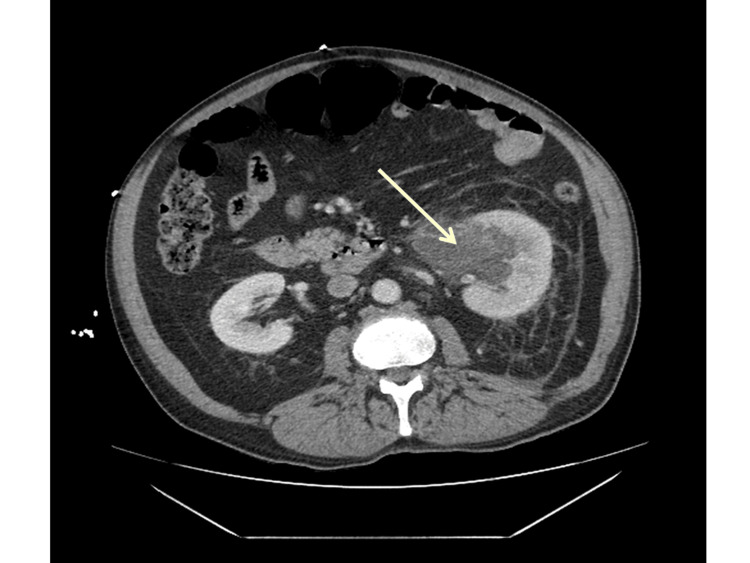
Contrast-enhanced CT (axial view) demonstrating a left renal mass (arrow), radiographically consistent with clear cell renal cell carcinoma.
